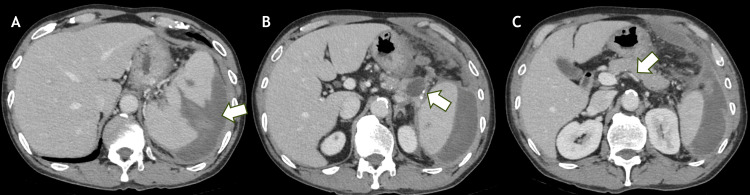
Contrast-enhanced axial computed tomography findings on admission (A) Axial contrast-enhanced computed tomography image demonstrating splenic laceration with associated perisplenic hematoma. (B) Axial computed tomography image showing a cystic lesion in the pancreatic tail adjacent to the splenic hilum. (C) Axial computed tomography image demonstrating narrowing/occlusion of the splenic vein anterior to the abdominal aorta. No active contrast extravasation is identified.
